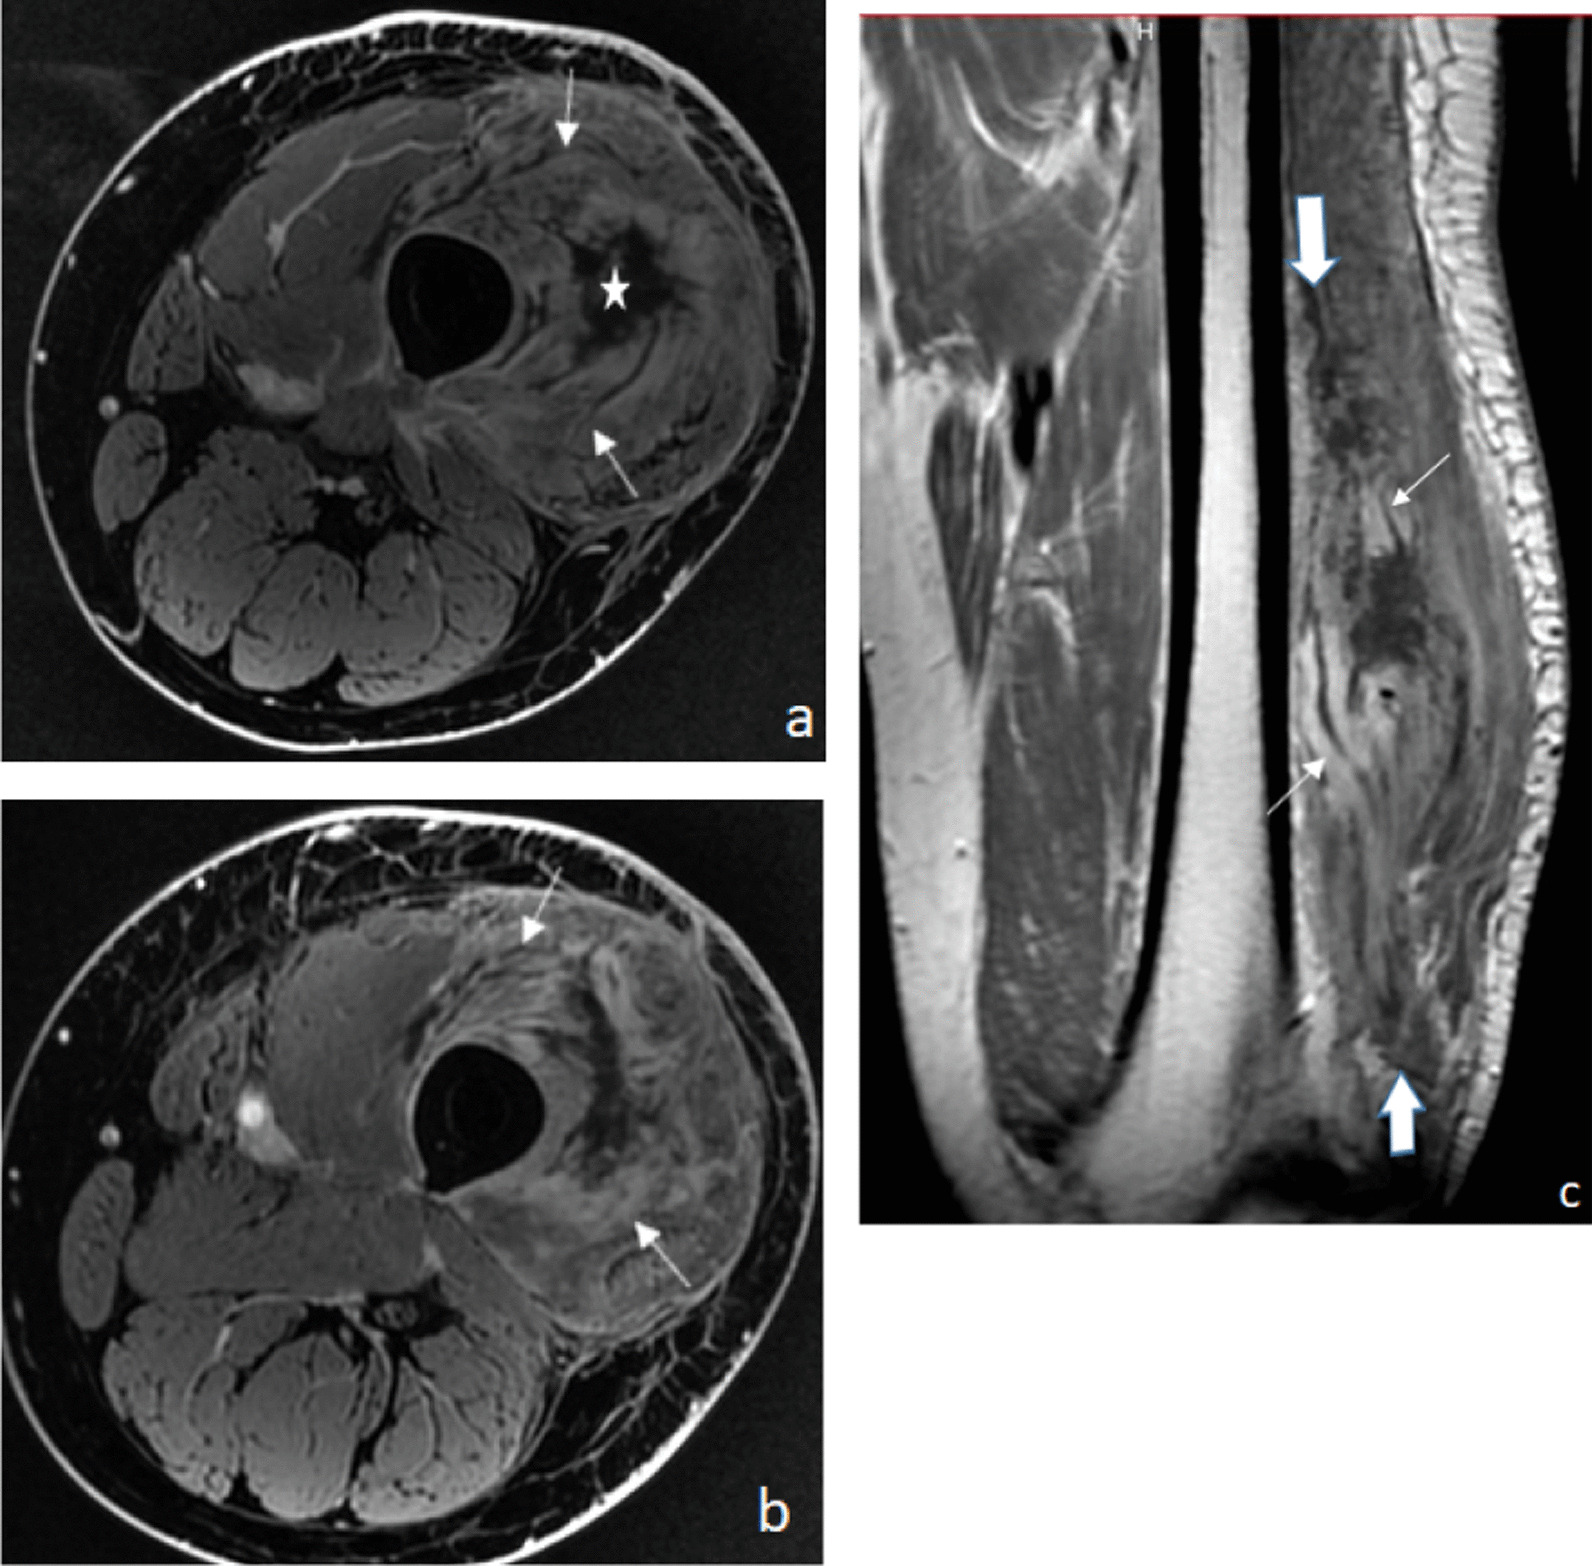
Early-stage axial fat-suppressed T2-weighted MR image (a) showing a marked, diffuse, high-signal edema of the soft tissue (arrows) circumscribing a central core (star). Gadolinium-enhanced fat-suppressed T1-weighted MR image (b) shows diffuse enhancement of the intermuscular fascia (arrows) without any nodular mass. Coronal T_1_-weighted MR image (c) shows extensive infiltration of the soft tissue (big arrows) with convergence of normal muscular fibers (fine arrows) through the central core. *MR* magnetic resonance
